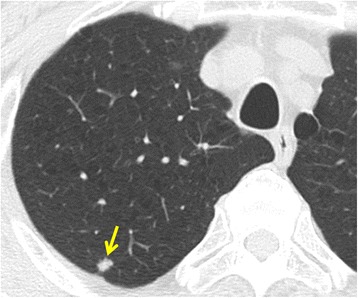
Chest computed tomography (CT) revealed a well-demarcated solid nodule in the right upper lobe adjacent to the pleura, which measured 1.0 cm in diameter
